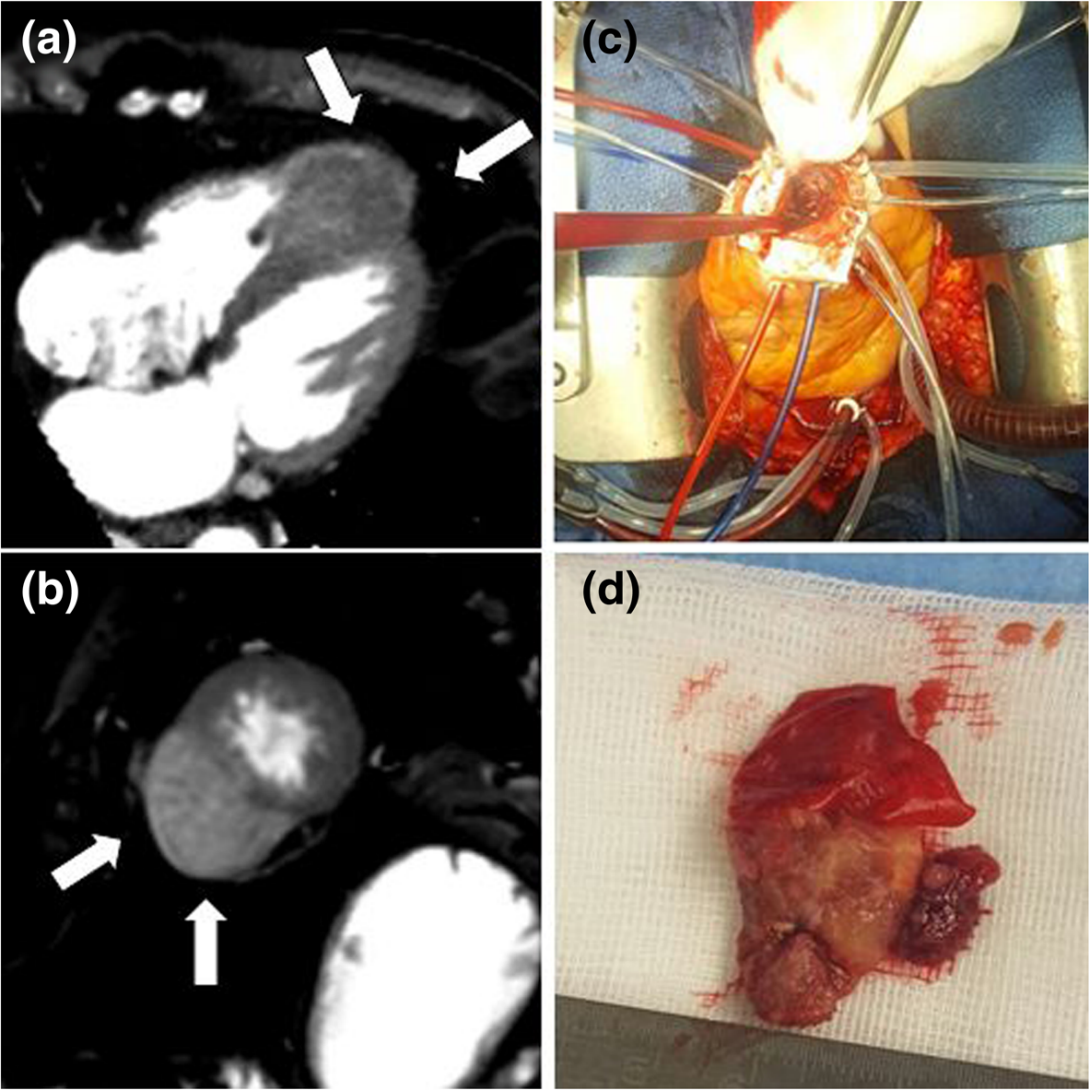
Preoperative imaging and intraoperative views of the metastatic tumor. a CT (256 Multislice Philips ICT, arterial phase, 4-chamber reconstruction images) and (b) MRI (Siemens Magnetom Prisma, 3 Tesla, T2 weighted single-shot TRUFI sequence) showing HCC metastasis infiltrating ventricular walls and septum, (c) intraoperative view showing apex with tumor exposed, (d) 27 × 27 × 12 mm section of tumor removed in toto
